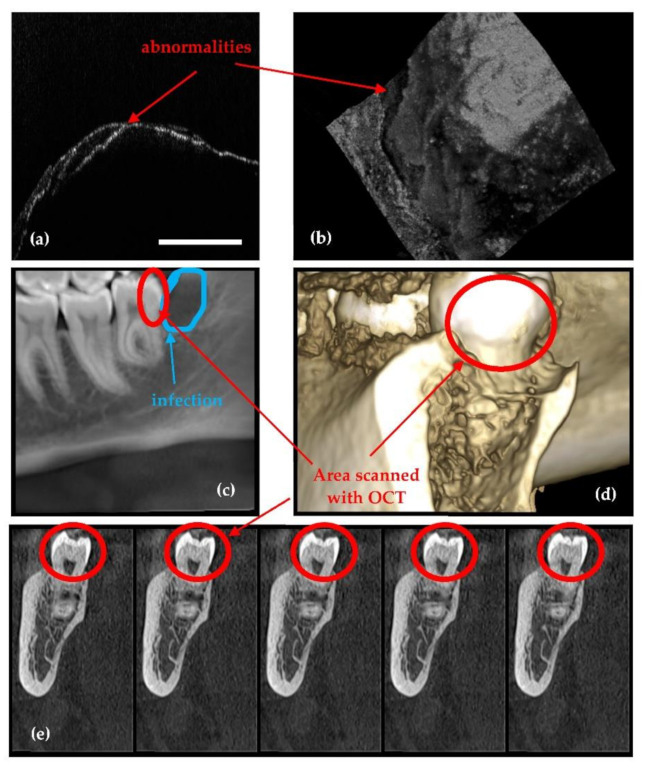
Example where OCT spots abnormalities on the enamel and dentine level of the tooth. (a) OCT B-scan; (b) OCT 3D reconstruction; (c) 3D CBCT axial view of the tooth; (d) 3D rendering of CBCT; (e) 3D CBCT sagittal view of the tooth (the latter taken at different, successive depths into the hard tissue). Scale: 1 mm.
